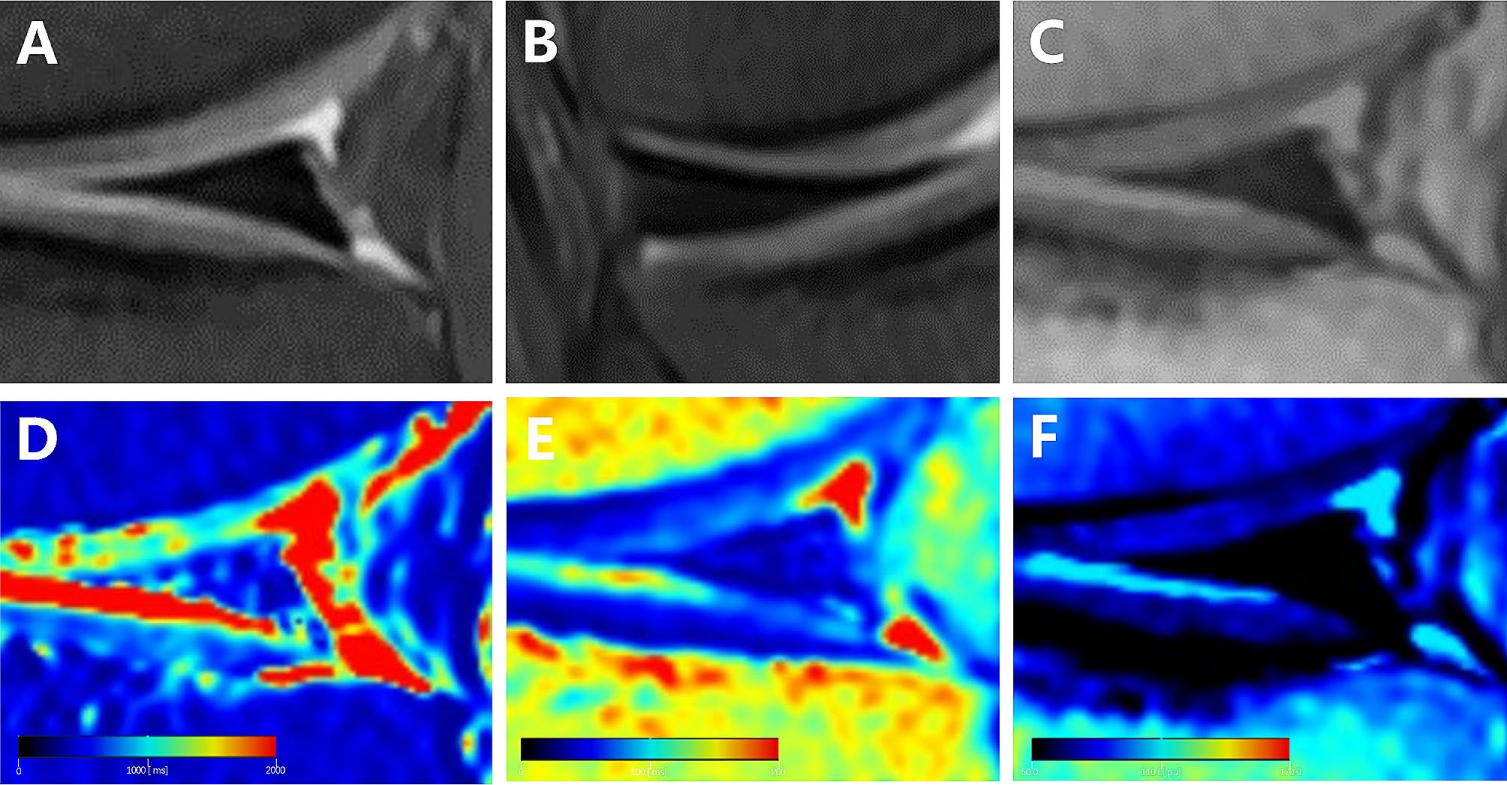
A 30-year-old male subject with posterior horn of the medial meniscus of left knee joint. A: Sagittal PDWI; B: Coronal PDWI; C: synthetic sagittal PDWI; D: synthetic sagittal T1 mapping; E: synthetic sagittal T2 mapping; F: synthetic sagittal PD mapping. There is no abnormal signal conventional MRI (A and B) and SyMRI (C and F) in the posterior horn of the lateral meniscus, but there is abnormal signal on SyMRI (blue color in the meniscus, D and E) within the meniscus
